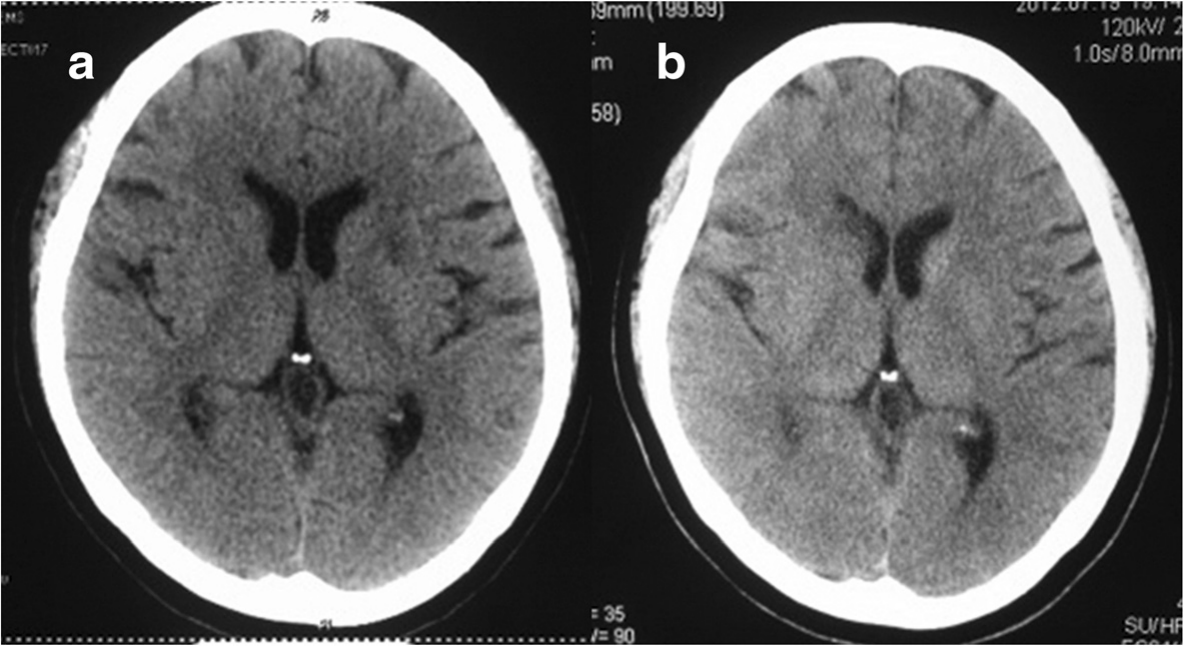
The patient’s cerebral computed tomographic scans. a Scan taken 9 days after the patient’s unconsciousness. b Scan obtained 19 days after the patient’s unconsciousness, showing improvement in her condition
